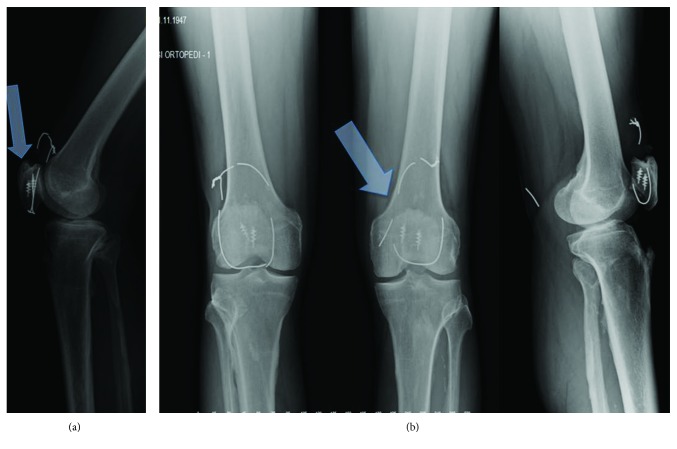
Bilateral knee X-rays after 8 years. Note the spur formations from the tibial tuberosity and patella (associated DISH) in the lateral view of the left knee ((b) right arrow). Bilateral cerclage wires have been broken ((a) left arrow).
